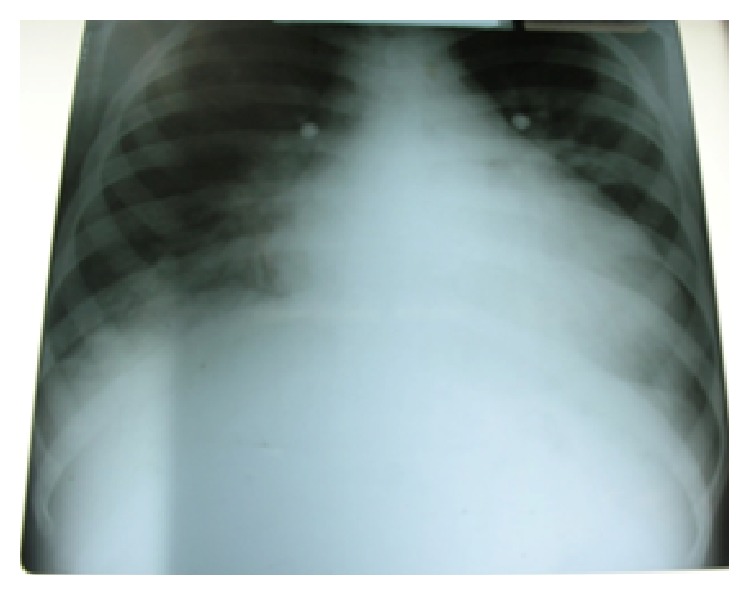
Chest X-ray showing the massive cardiomegaly.
